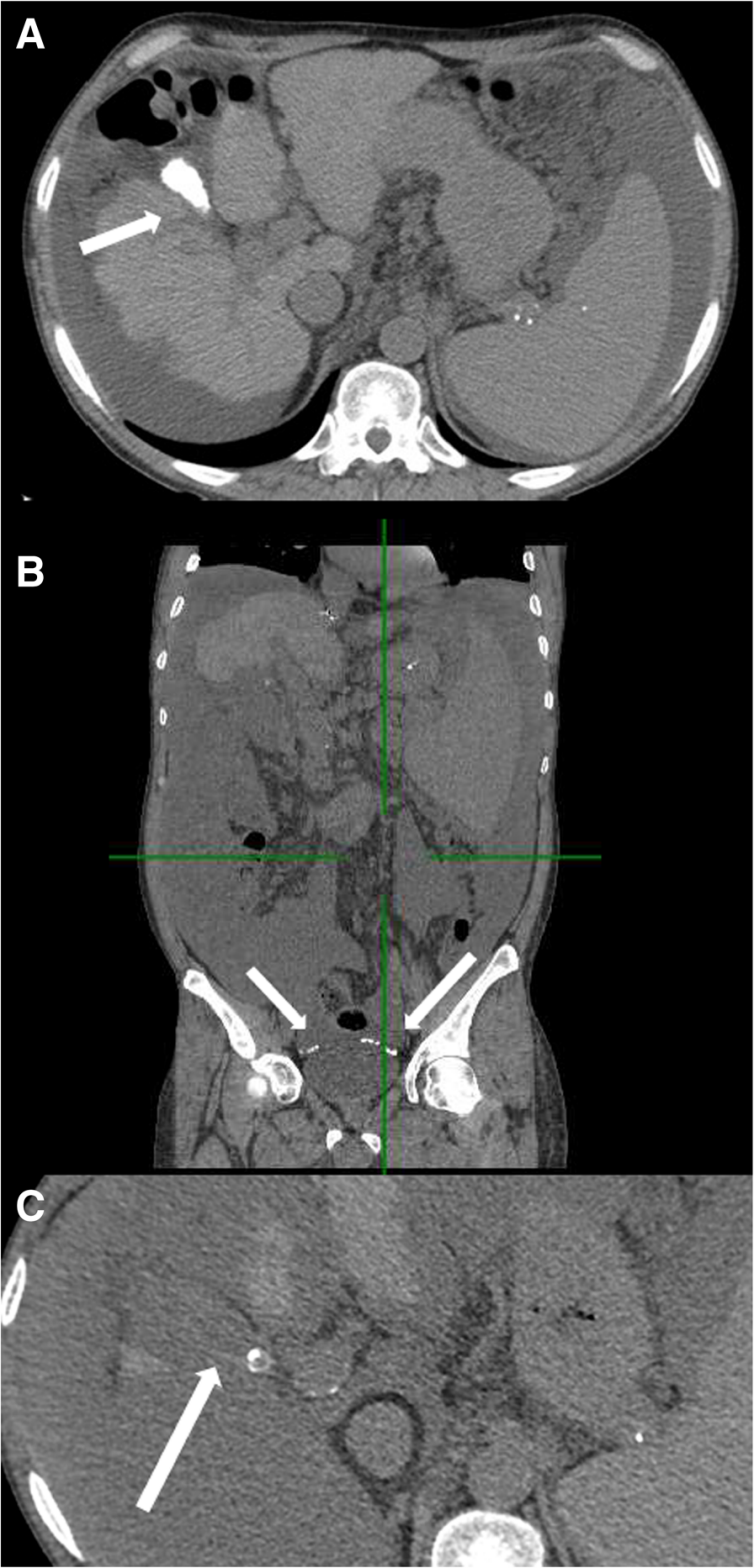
a Abdominal CT performed a month before: scleroatrophic cholecystitis whose lumen is completely occupied by lithiasic formations (arrow). b Abdominal CT performed in urgency: numerous radiopaque elements were evident in the pelvis (arrows) alongside dense ascites. c Abdominal CT performed in urgency: scleroatrophic gallbladder bearing only one stone inside and irregularity along the wall but no frank interruption
